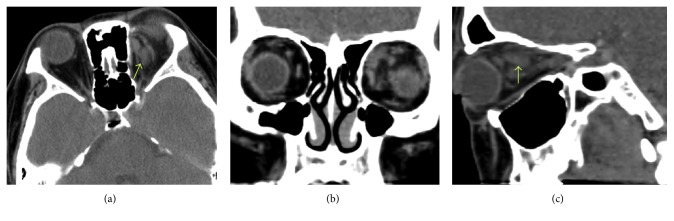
CT scan of case 2. (a) Axial, (b) coronal, and (c) sagittal view. There was retrobulbar infiltration with possible abscess formation (arrows). The sinuses were clear.
